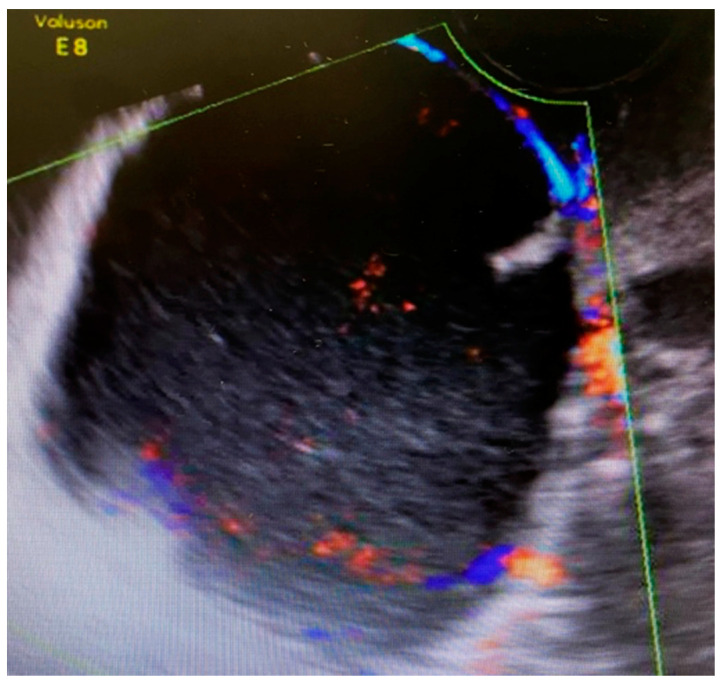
Ultrasound scan compatible with complex cystic formation.
